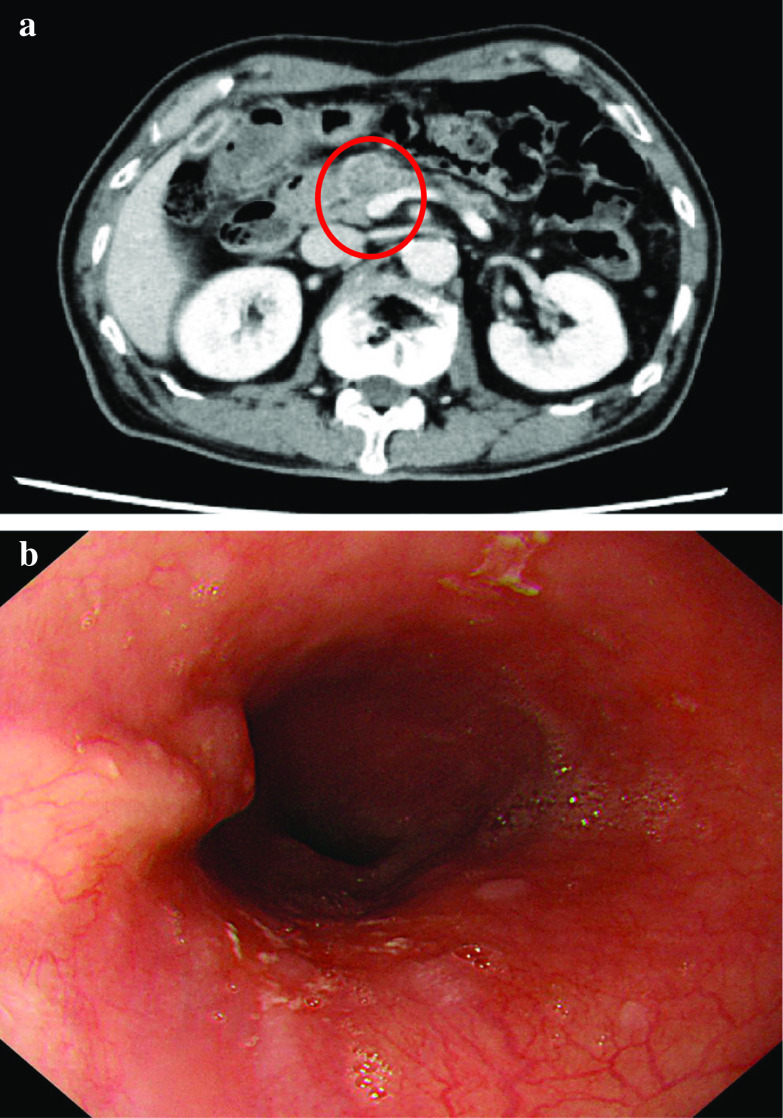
Therapeutic assessments after neoadjuvant chemotherapy for treatment of pancreatic cancer. a Computed tomography showed regression of the pancreatic tumor (red circle) in size from 26 to 18 mm after neoadjuvant chemotherapy. b The regression of esophageal cancer was endoscopically verified for its invasion into the muscularis mucosae after neoadjuvant chemotherapy for treatment of pancreatic cancer
